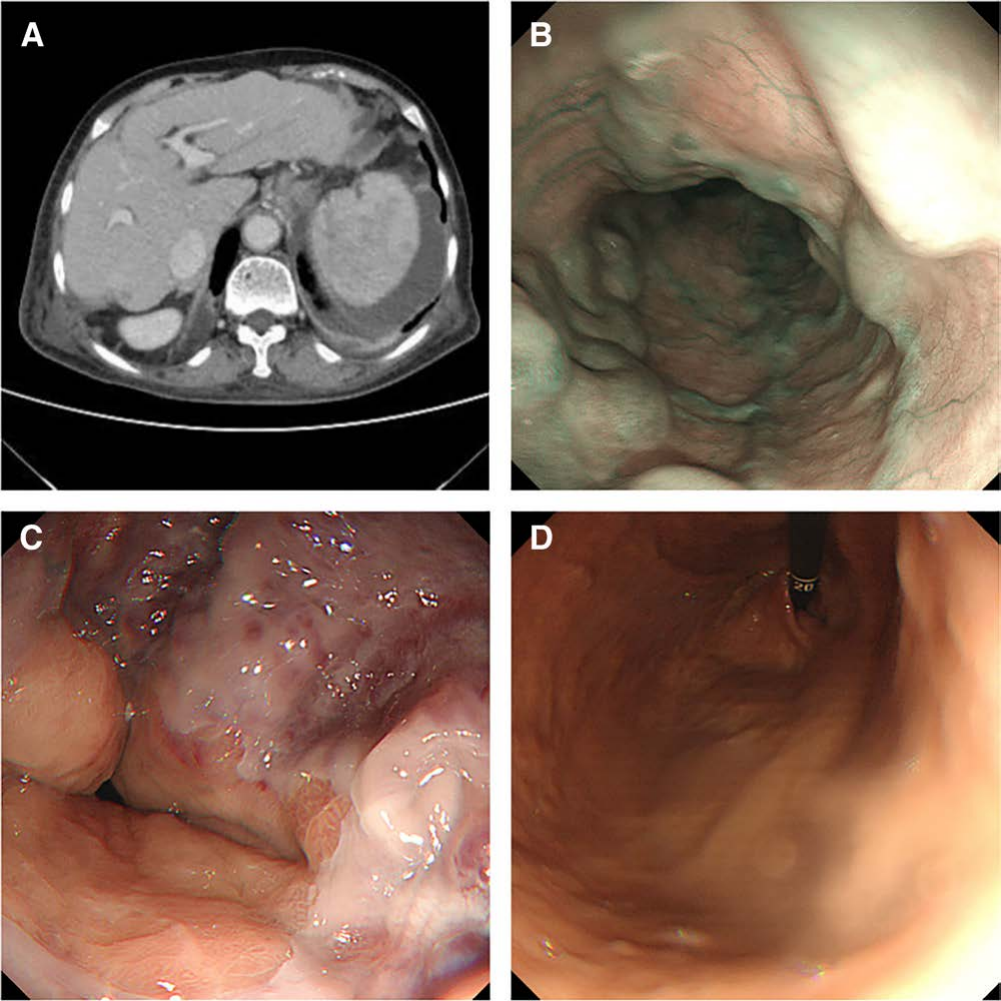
(A) Abdominal CT (2024.03.14) showed ascites, altered liver morphology, mild splenomegaly and portal hypertension. (B) Gastroscopy indicated severe esophageal varices. (C) Red color sign. (D) Image of gastric fundus.
